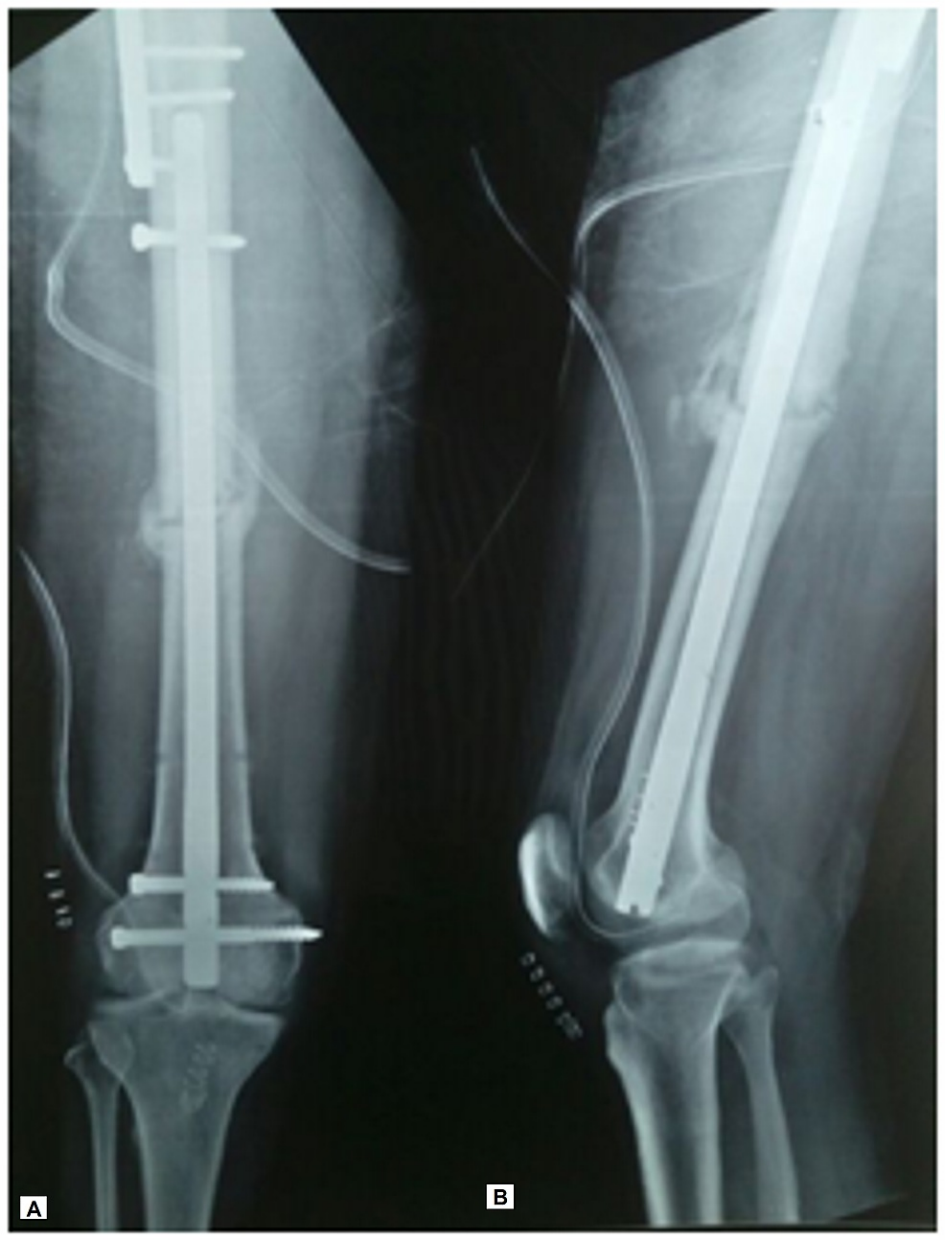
(A, B) Immediate postoperative radiograph showing fixation of femoral shaft using reamed retrograde nail
